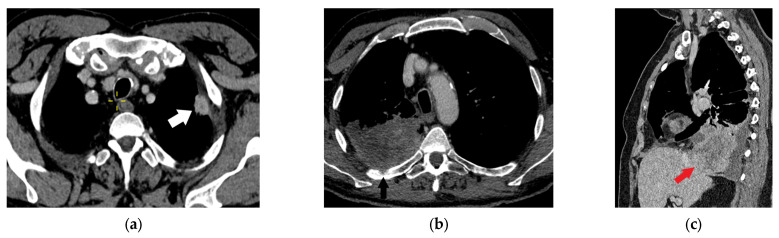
Parietal pleura, chest wall, and diaphragm invasion: Axial contrast-enhanced chest CT image shows lung cancer in the upper-left lobe (white arrow) invading the parietal pleura and extrapleural fat (T3 category, (a)). Rib erosion (black arrow) by upper-right lobe lung cancer is a reliable sign of chest wall invasion (T3 category, (b)). A sagittal multiplanar reformatted image shows an invasion of the diaphragm (red arrow) by the lower-right lobe lung cancer (T4 category) with associated pleural effusion (c).
